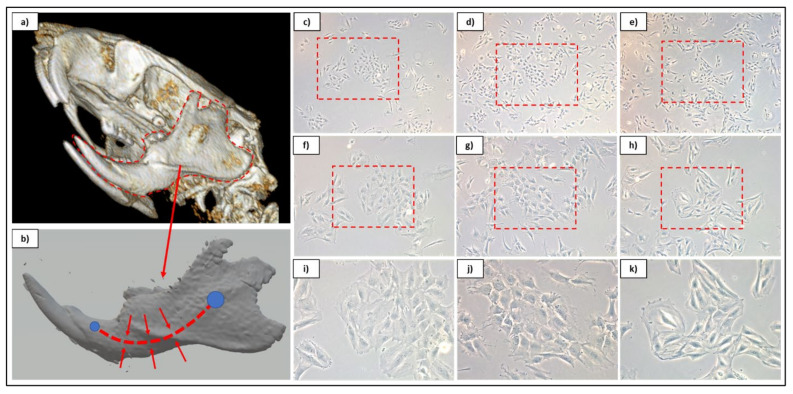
Overview graphic for dental pulp site on CT rendering (a) and reconstructed mandibular 3D model (b). Cultured cell isolates from αMEM-cultured cells from both enzymatic (c,f,i) and tissue outgrowth (d,g,j) methods and DMEM/F12-cultured cells using tissue outgrowth method (e,h,k). Phase contrast culture images are displayed at 5× (c–e), 10× (f–h), and 20× (i–k) magnifications.
